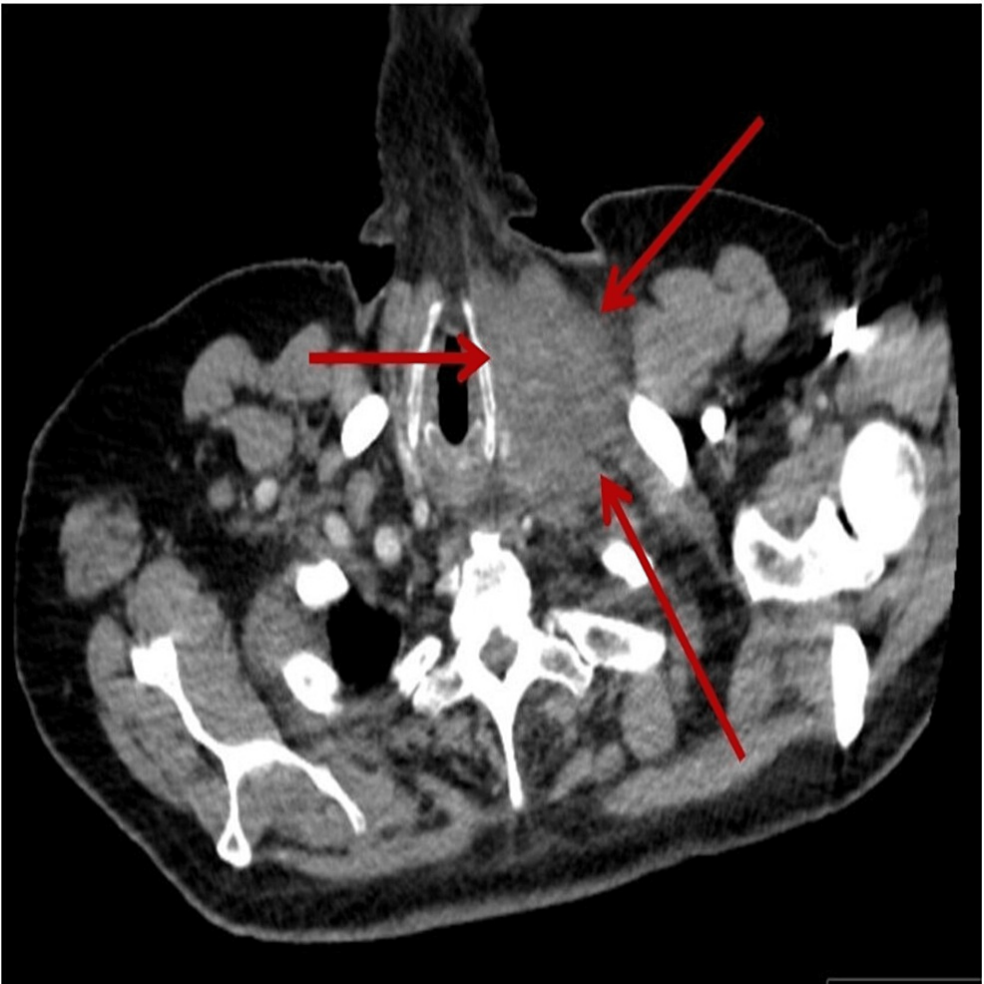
CT chest axial view showing a large ill-defined left supraclavicular mass (area pointed at by the three arrows)
